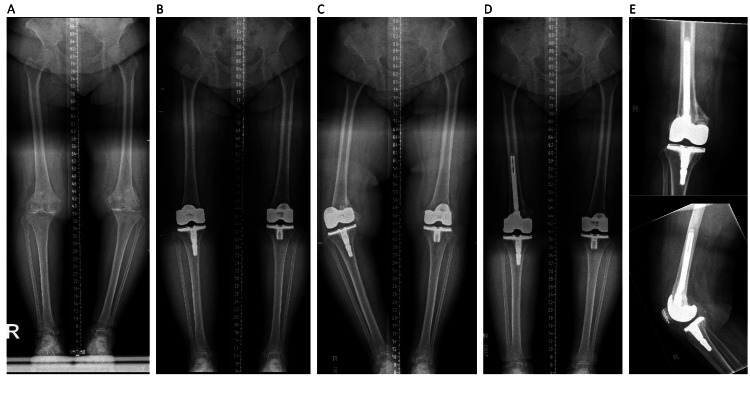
Patient 6 serial radiographs showing the alignment view and post-revision AP and lateral radiographs in chronological order from left to right A: preoperative long leg film, B: two weeks postoperative long leg film, C: one year post FCIF-TKA long leg film, D: two weeks post revision long leg film, E: two weeks post revision AP and lateral radiographs AP: anterior-posterior, FCIF-TKA: femoral condyle insufficiency fractures following total knee arthroplasty
